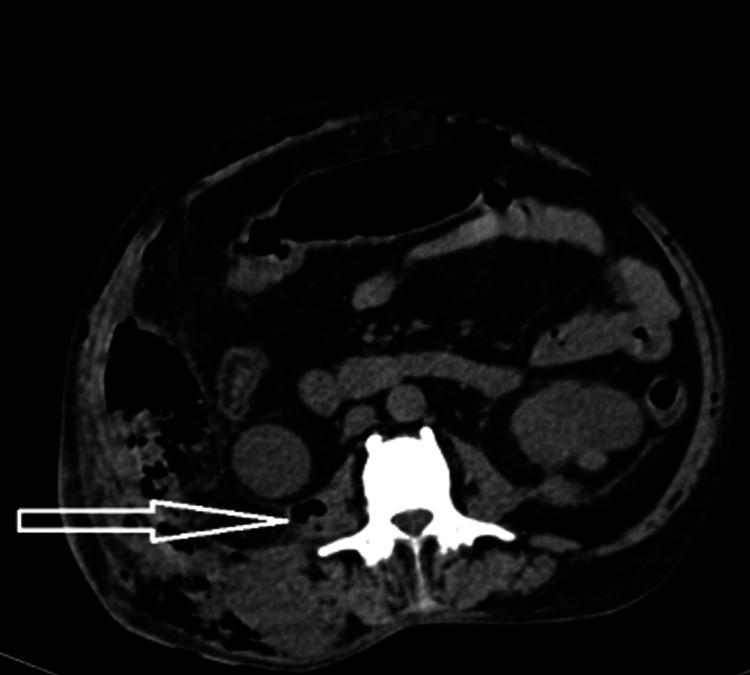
Contrast-enhanced CT abdomen axial section showing a collection and air focus along the right psoas muscle CT: computed tomography
